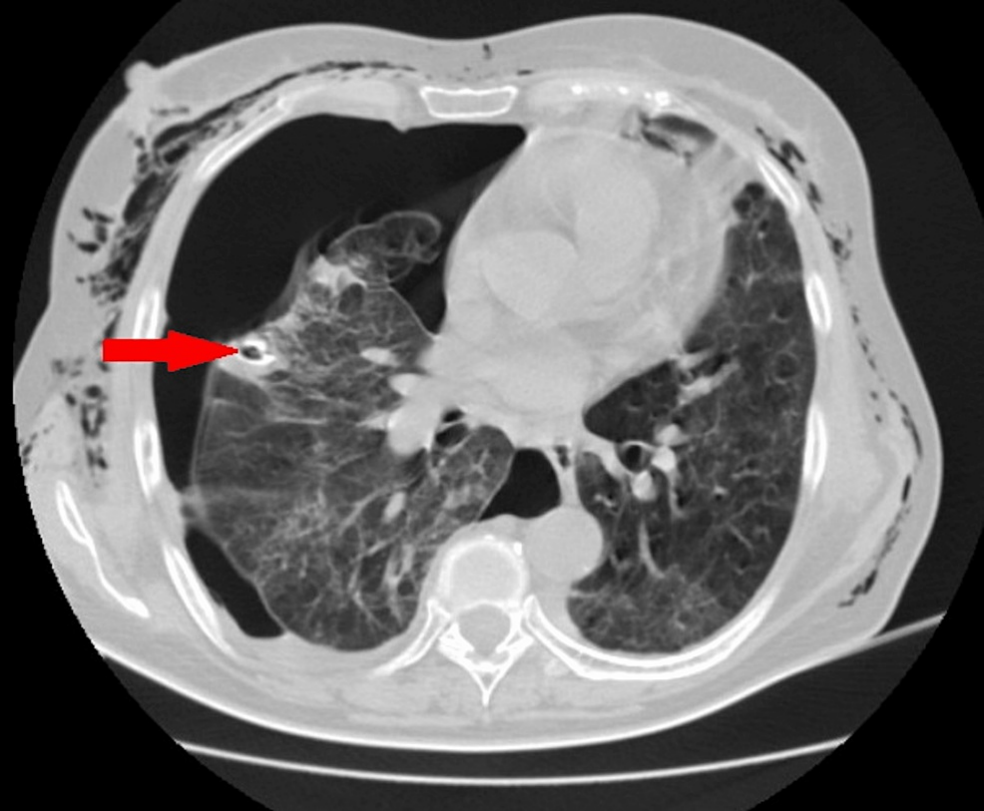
Contrast-enhanced chest CT (4th day of tube thoracostomy) showing a cavitary mass in the right upper lobe (shown by arrow).
